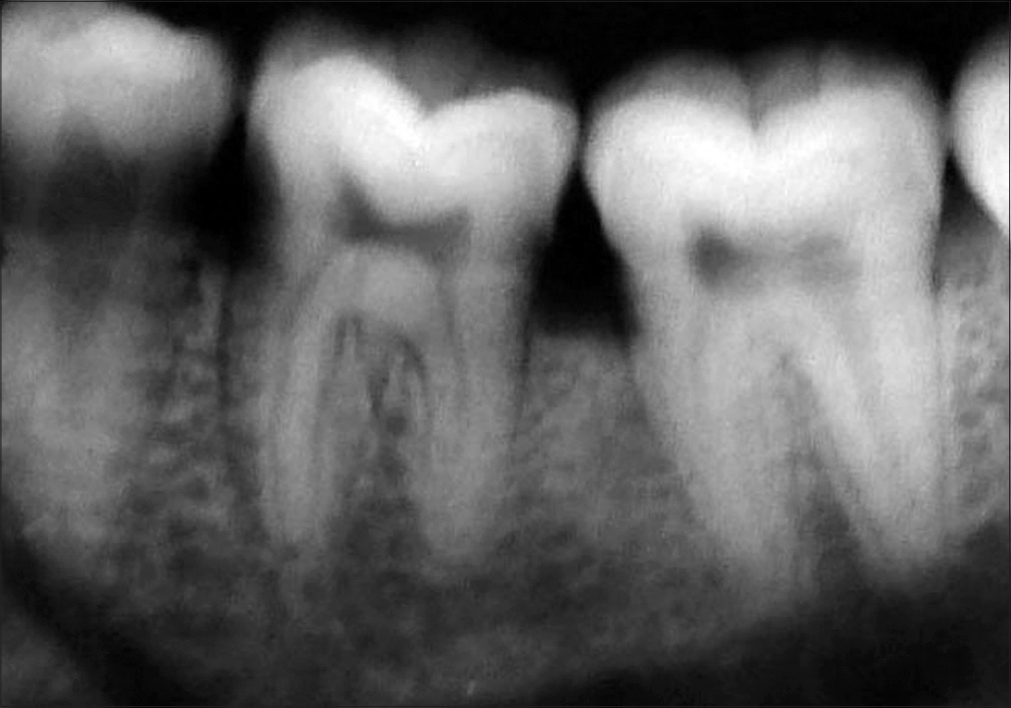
Preop radiograph (left side)
